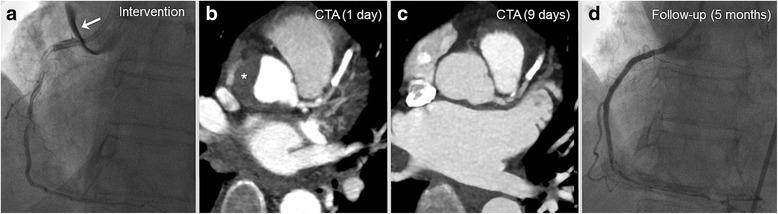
Dunning Dissection type II. Patient with 3-vessel coronary artery disease and PCI of the LAD and 90% long-segment stenoses of the RCA. a After intubation of the RCA with an AL1 a spiral winded DD occurred with failed rewiring. b cCTA confirmed the extensive haematoma. c The patient underwent a conservative wait and see with closed meshed cCTA with four cCTA within 9 days and d final satisfactory result. cCTA, coronary CT angiography; PCI, percutaneous coronary intervention; RCA; right coronary artery
